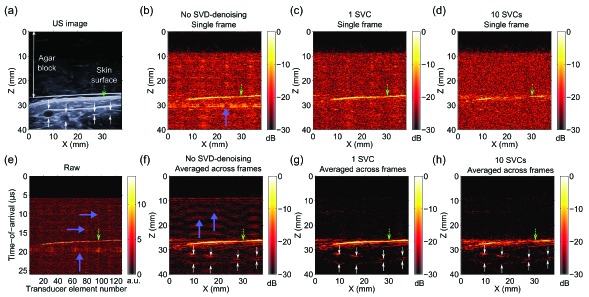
Laser-induced noise identification with singular value decomposition (SVD) in photoacoustic images acquired from a human forearm *in vivo.* These data, which were acquired with an L14-5/38 ultrasound (US) imaging probe, were co-registered with a B-mode pulse-echo US image (a). With B-mode US imaging, four superficial blood vessels were apparent (top and bottom of each vessel indicated with white arrows). Beneath the overlying agar block, there was a thin layer of US gel before the skin surface (dashed green arrow). Laser-induced noise manifested as prominent bands [(b), thick purple arrow] with a magnitude comparable to that of signals from the skin surface and larger than that of signals from blood vessels (not apparent). Laser-induced noise identification with 1 singular value component (SVC) and subsequent denoising yielded a substantial improvement in photoacoustic image quality, with the noise band absent (c). When 10 SVCs were used, the magnitude of the signal from the skin surface was reduced (d). In the raw radiofrequency data, vertical and horizontal noise bands were apparent [(e), prominent examples indicated with thick purple arrows]. When averaging across 31 PA images was performed, signals from the blood vessels were apparent but laser-induced noise across the image (prominent examples indicated with thick purple arrows) had comparable magnitudes (f). When averaging across PA images and SVD-denoising with 1 SVC were performed, the laser-induced noise was absent and signals from the blood vessels were clearly visible (g). The signals from the skin surface and the blood vessels were smaller when 10 SVCs were used (h). All reconstructed photoacoustic images were normalised to their maximum values and displayed on logarithmic scales with the same dynamic range (30 dB). The raw data in (e) is displayed on a linear scale.
